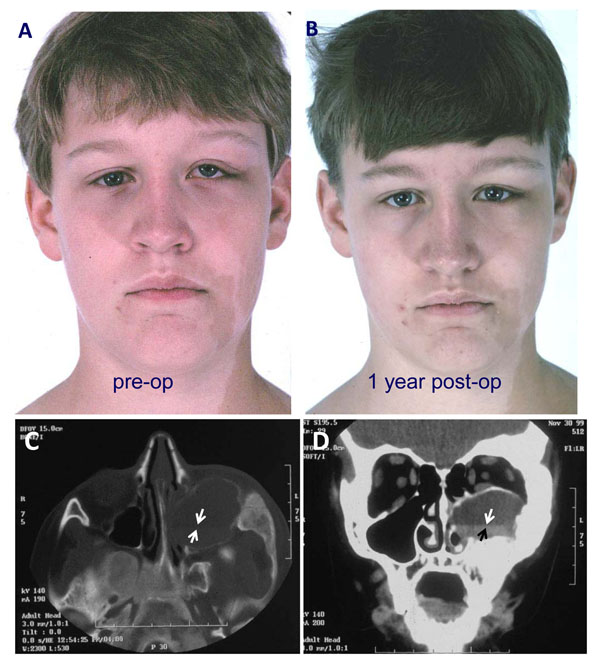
Fibrous dysplasia with a secondary aneurysmal bone cyst (ABC). A) The patient with a history of MAS complained of visual changes. Worsening asymmetry of the left eye and face was noted, and an on examination he was noted to have vertical dystopia of the orbit in the preoperative photograph. He was found to have a rapidly growing ABC within FD and underwent immediate resection and decompression of the ABC. B) The asymmetry and symptoms resolved after surgery. Note the classic café au lait spots of the left face and neck region as part of the triad of MAS. C&D) Preoperative CT images of the patient in A showing the FD lesion and associated ABC. Note the fluid/fluid level diagnostic of an ABC (arrows). The association of an ABC often results in aggressive behavior and rapid enlargement of the FD lesion with displacement of adjacent structures, in this case, the eye.
